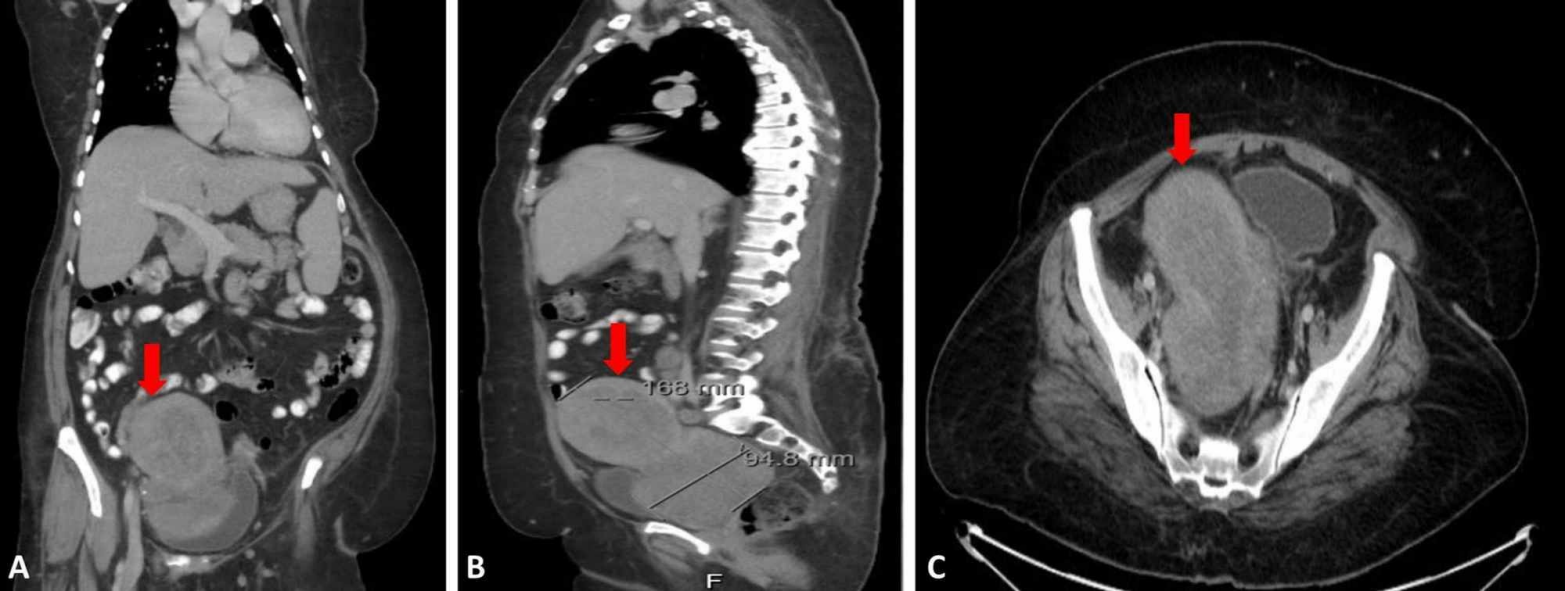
Coronal (A), sagittal (B) and axial (C) CT scans demonstrating a large uterine mass (red arrow) extending into the upper vagina and abutting the rectum and urinary bladder.
